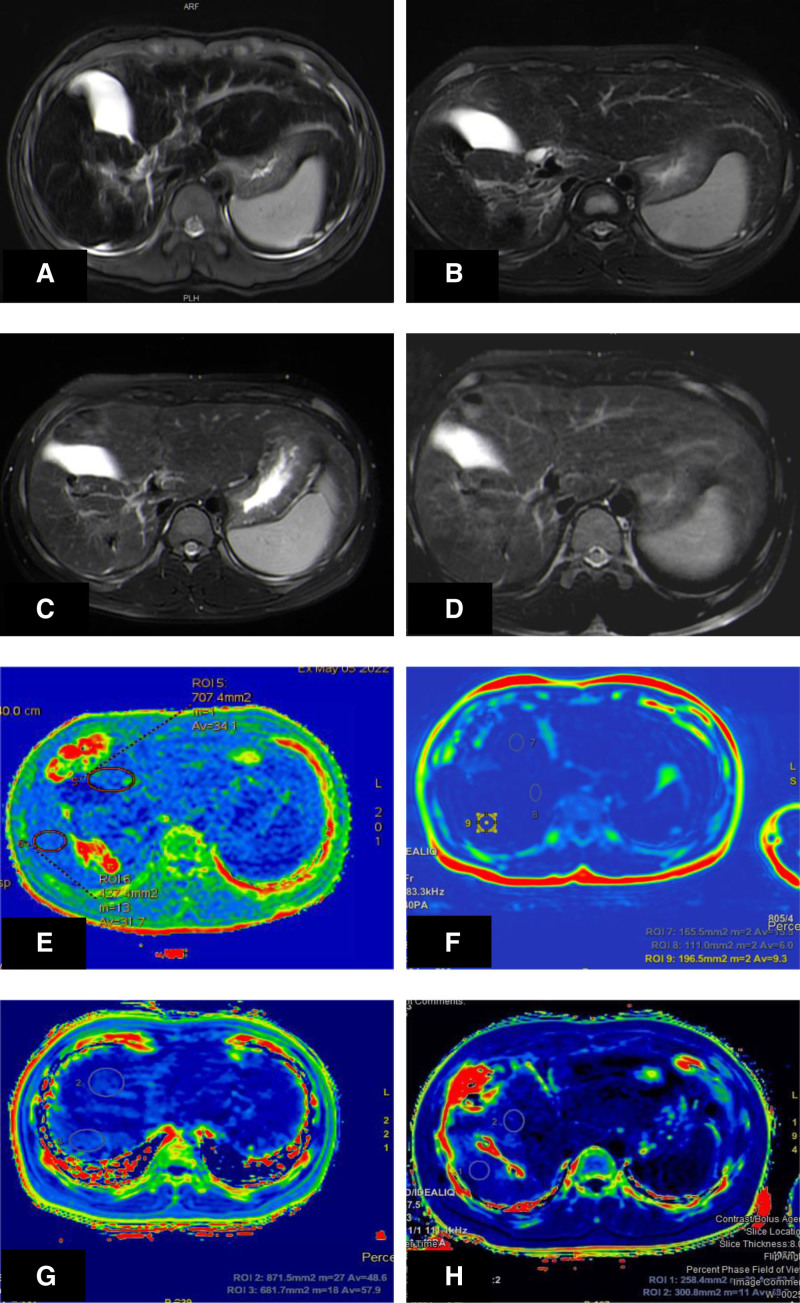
Short-axis plane of liver by T2-weighted turbo-spin echo sequence (the more the iron content, the lower the signal of the liver, and the lower the value of T2). (A) Liver MRI at initial diagnosis. (B) Liver MRI at 24 weeks of treatment. (C) Liver MRI at 48 weeks of treatment. (D) Liver MRI at 106 weeks of treatment. The results showed that the liver iron deposition was gradually improved. (E) Liver MRI measurements of R2* at 106 weeks of treatment. (F) Liver MRI measurements of R2* at 142 weeks of treatment. (G) Liver MRI measurements of R2* at 162 weeks of treatment. (H) Liver MRI measurements of R2* at 178 weeks of treatment. MRI = magnetic resonance imaging.
